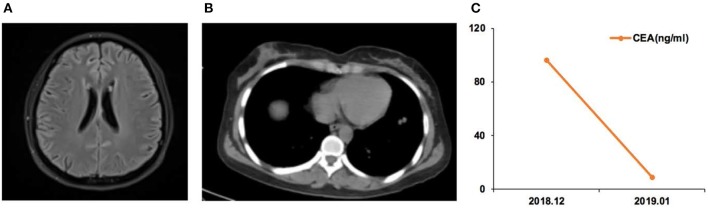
Case 05 was a male of 71 years of age, with lung adenocarcinoma but without smoking history. (A) Cerebellar vermis, bilateral cerebral hemispheres, and pia meninges shoed abnormal enhancement on magnetic resonance imaging. Leptomeningeal metastasis (LM) was considered. (B) Chest computed tomography revealing the primary lung lesion. (C) Carcinoembryonic antigen (CEA) levels before and after osimertinib treatment.
